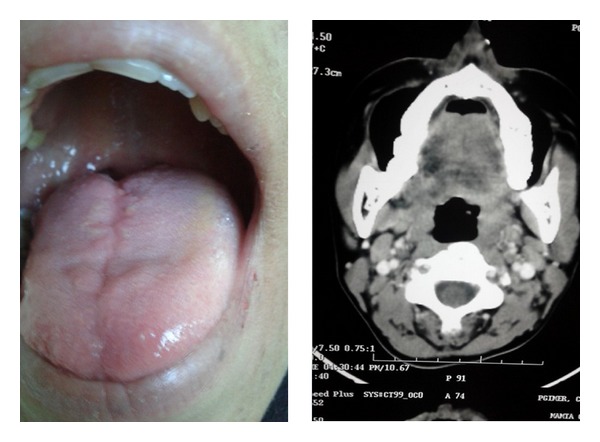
Appearance of tongue after 2 months following surgery and postoperative computed tomography.
